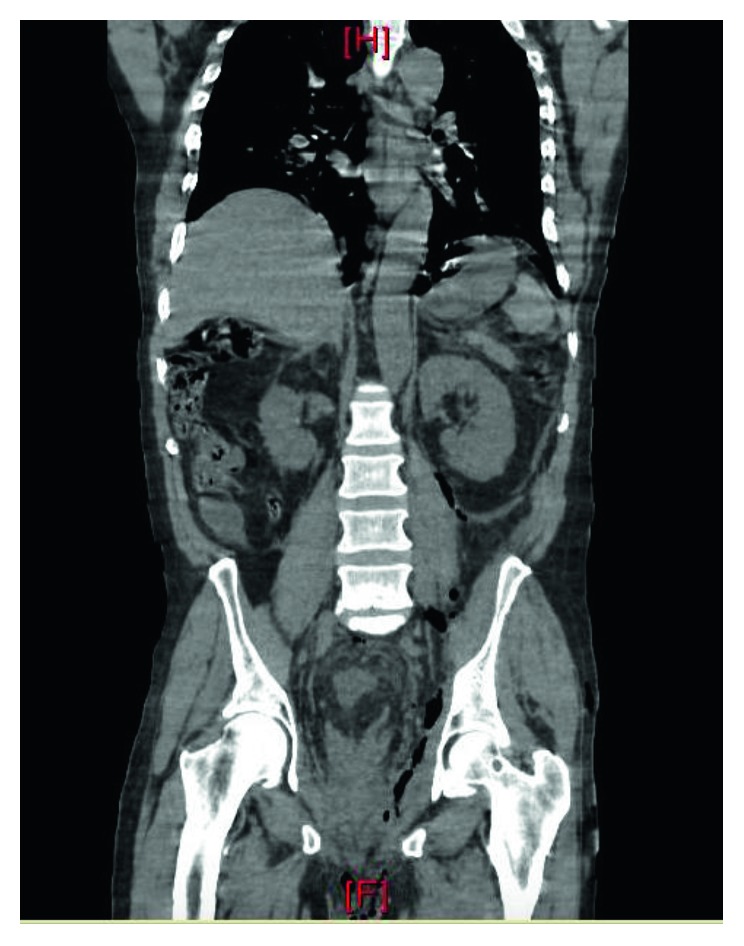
Coronal CT image showing gas tracking from left perirectal area superiorly into the left retroperitoneum to the level of the medial and inferior left kidney.
